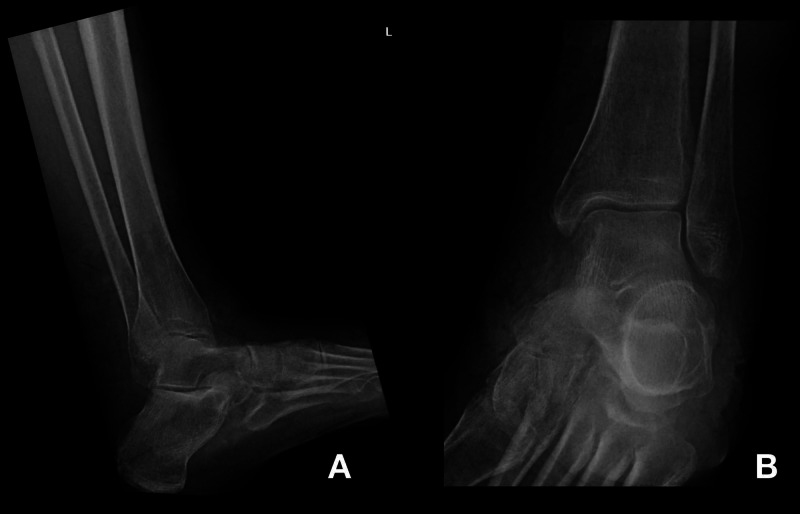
Plain radiograph of lateral (A) view and medial (B) view of the left ankle. No acute fracture or dislocation. Diffuse soft tissue swelling and subtle cortical erosions involving the medial aspect of the navicular bone as may be seen in osteomyelitis.
